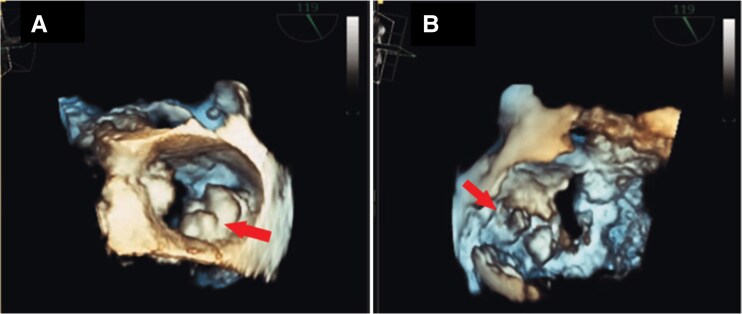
Transoesophageal echocardiography findings. 3D multi-planar reconstruction of the mitral: An En face view valve (*A*) and a ventricular view (*B*) showing extensive round to oval well delineated lobulated mass (arrows) extended along the posterior annulus, centred on the P1−P3 scallops with extension toward the posteromedial commissure and occupying the 3–5 and 7–9 o’clock positions.
